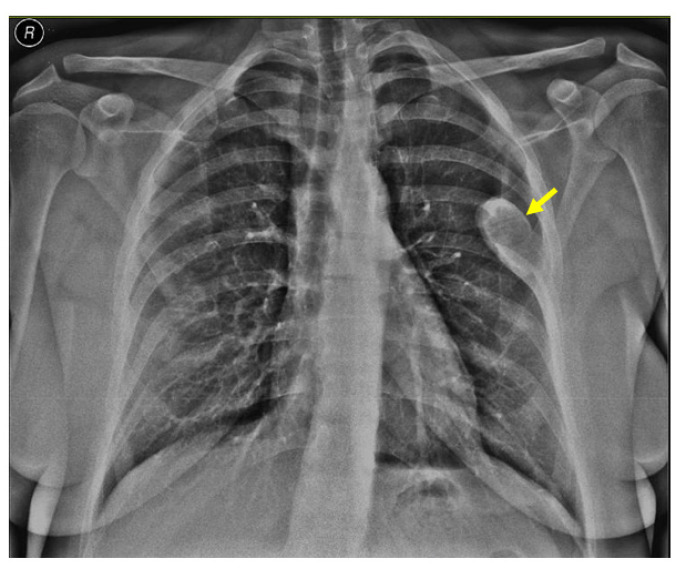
X-ray showing a brown tumor: a single, oval, well-shaped lesion of 6.4 by 2.7 cm in the lateral half of the left posterior sixth rib (yellow arrow).
